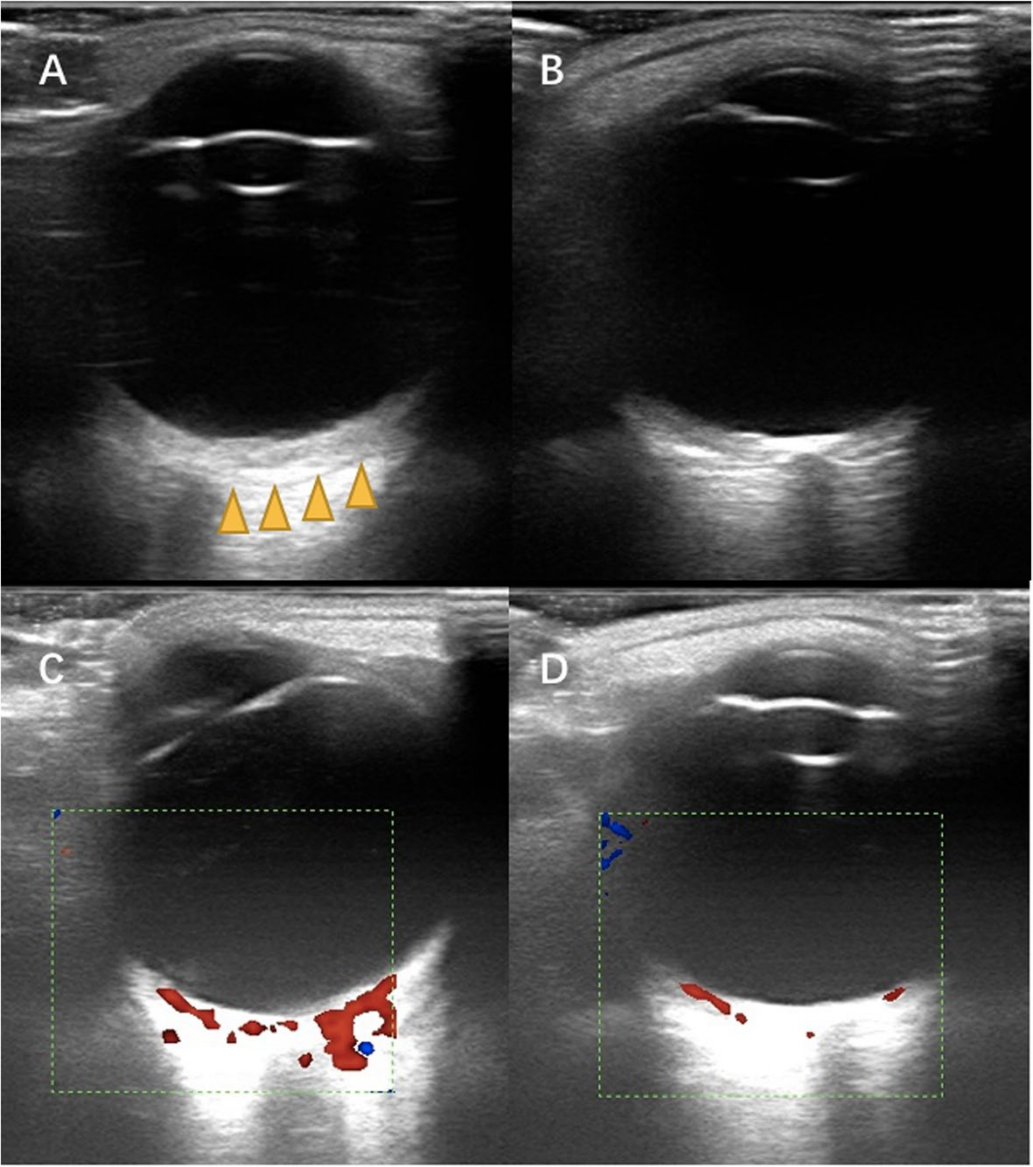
The ocular B-scan ultrasonography showed diffuse choroidal haemangiomas. Echo-enhanced thicker choroid with rich blood-flow signal in A (the yellow arrow) and C. The normal choroidal thickness and blood-flow signal in B and D
